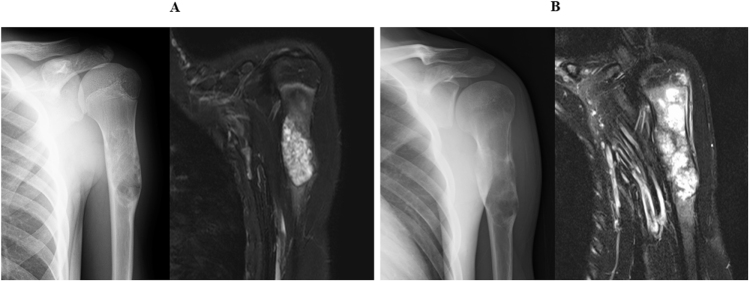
Radiographic and MRI findings at the initial visit and after 2 years of follow-up. (A) Plain radiograph and MRI at the initial presentation. (B) Follow-up imaging 2 years later, showing interval changes.
